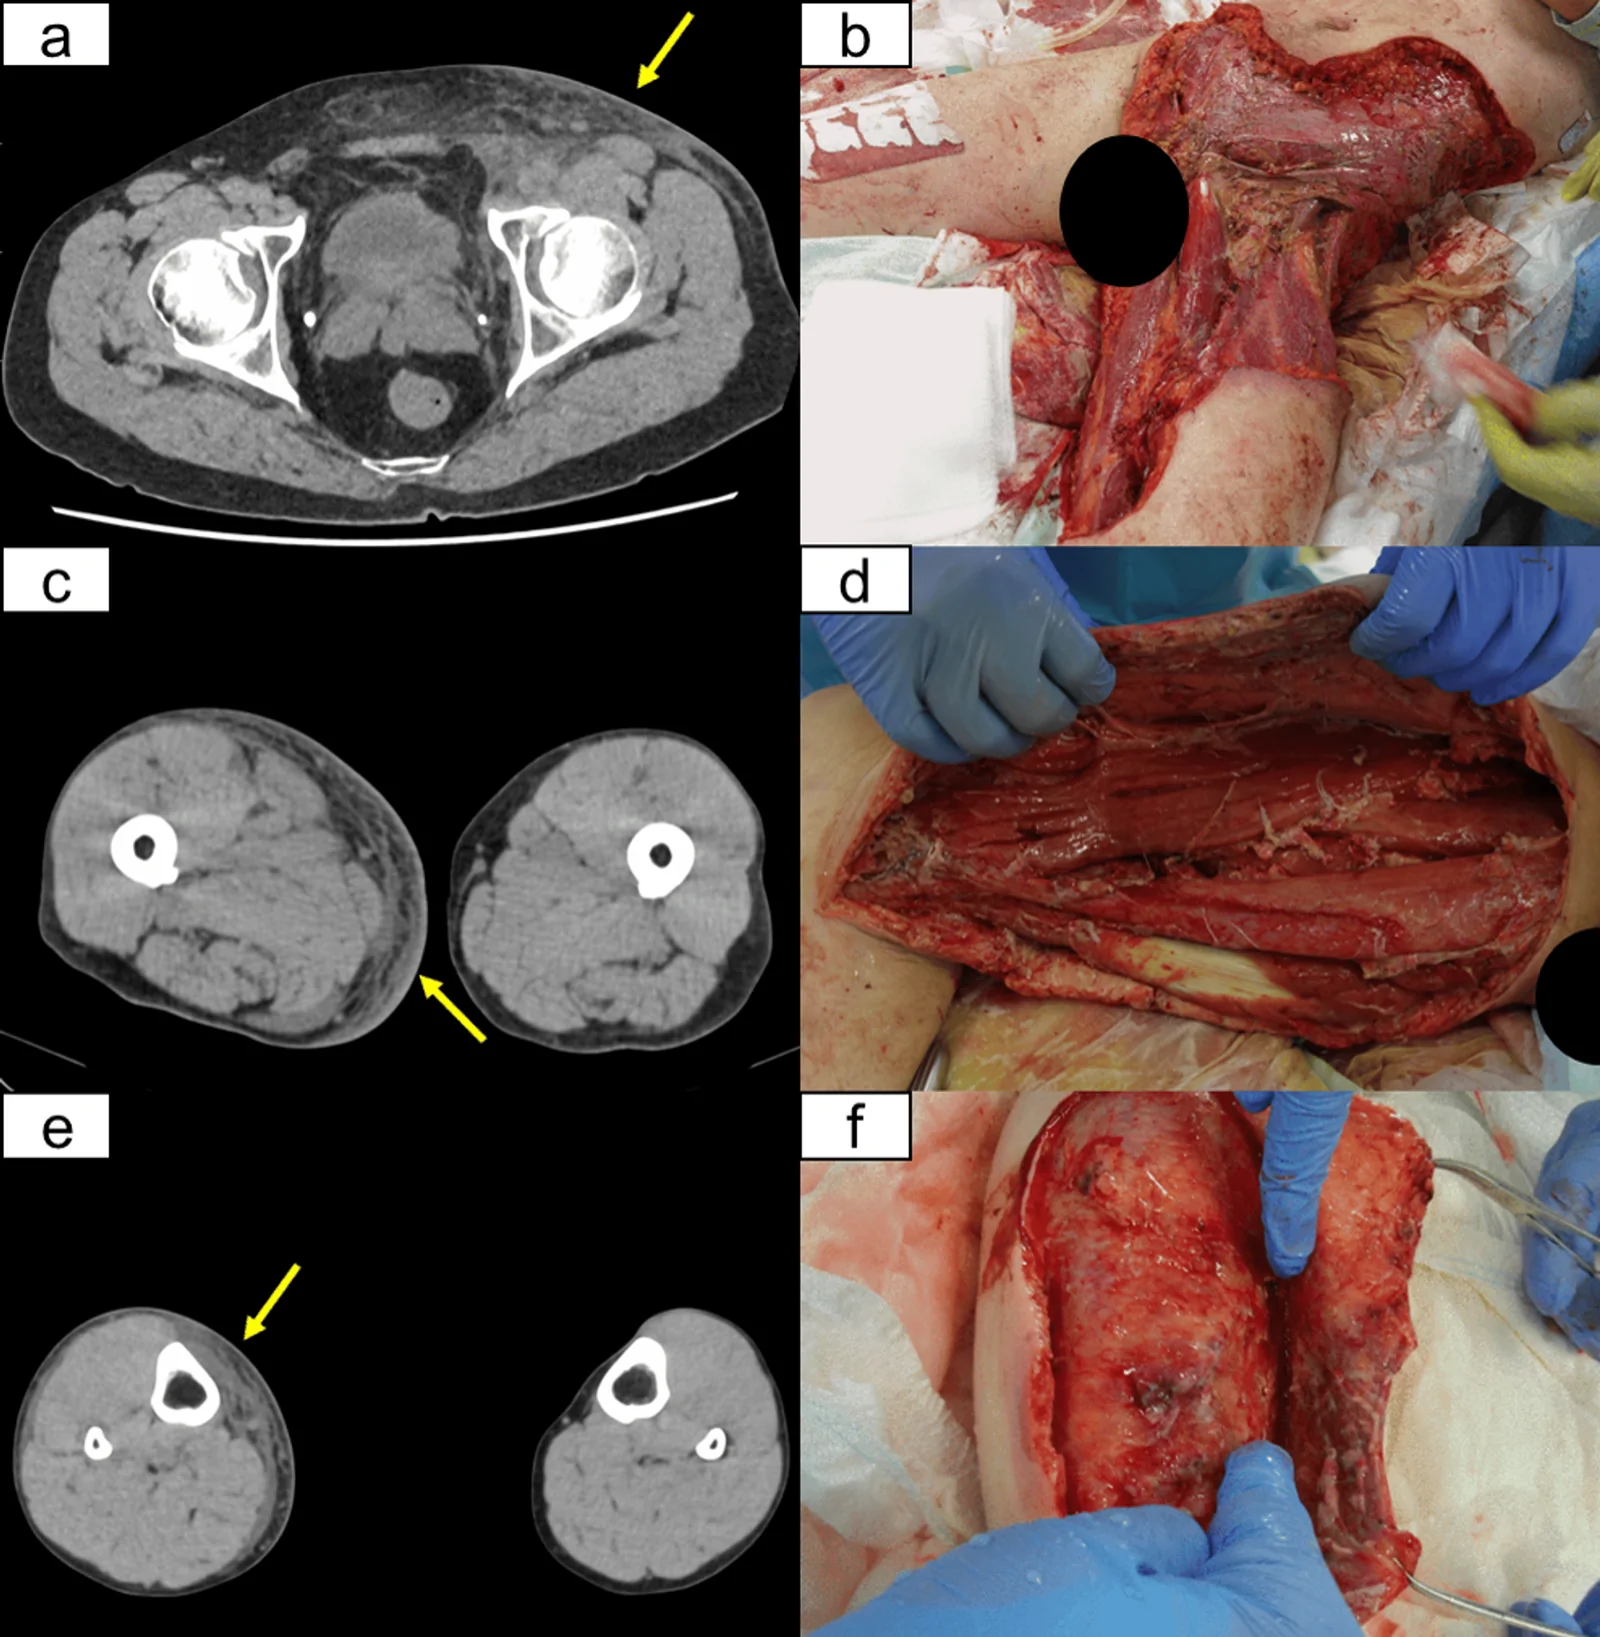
Findings of CT and wounds during emergent debridement on day 1 in each case. (1) CT findings of Case 1 (a). Subcutaneous fat stranding from the anterior to medial aspect of the left thigh. (b) Findings of the wound during the debridement in Case 1. Necrotic subcutaneous tissue, muscle, and fascia were incised across the left flank, bilateral lower abdomen, left anterior thigh, and bilateral spermatic cords and testes. (2) CT findings of Case 2 (c). Subcutaneous fat stranding and marked fascial thickening in the right thigh without gas. (d) Findings of the wound during the debridement in Case 2. Necrotic subcutaneous tissue, muscle, and fascia extending from the right proximal thigh to the right popliteal fossa were incised. (3) CT findings of Case 3 (e). Subcutaneous fat stranding and edema in the anteromedial right lower leg. (f) Findings of the wound during the debridement in Case 3. Necrotic subcutaneous tissue, muscle, and fascia were incised in the anterior aspect of the right lower leg.
